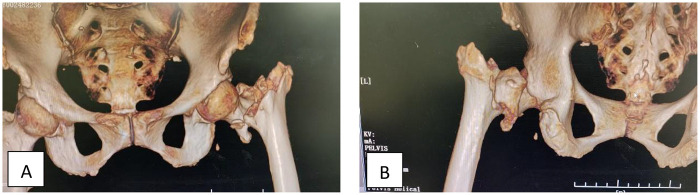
Preoperative 3D CT frontal (A) and dorsal (B) views show a simple two-part fracture of the trochanteric region without comminution (AO/OTA type 31A1.2). The fracture line extends from the greater trochanter along the trochanteric line to the medial lesser trochanter, bisecting the lesser trochanter into two parts.
